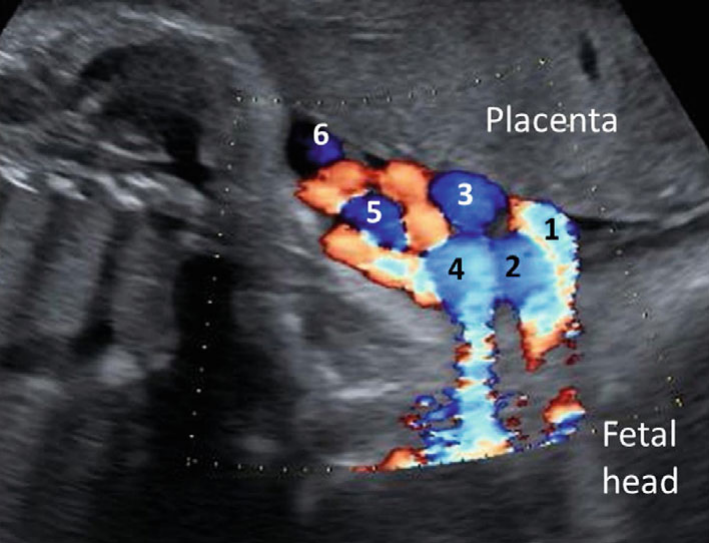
Coronal imaging of the fetal neck with color Doppler at 34 weeks of gestation. Numbers on the color Doppler show sextuple loops of nuchal cord.
